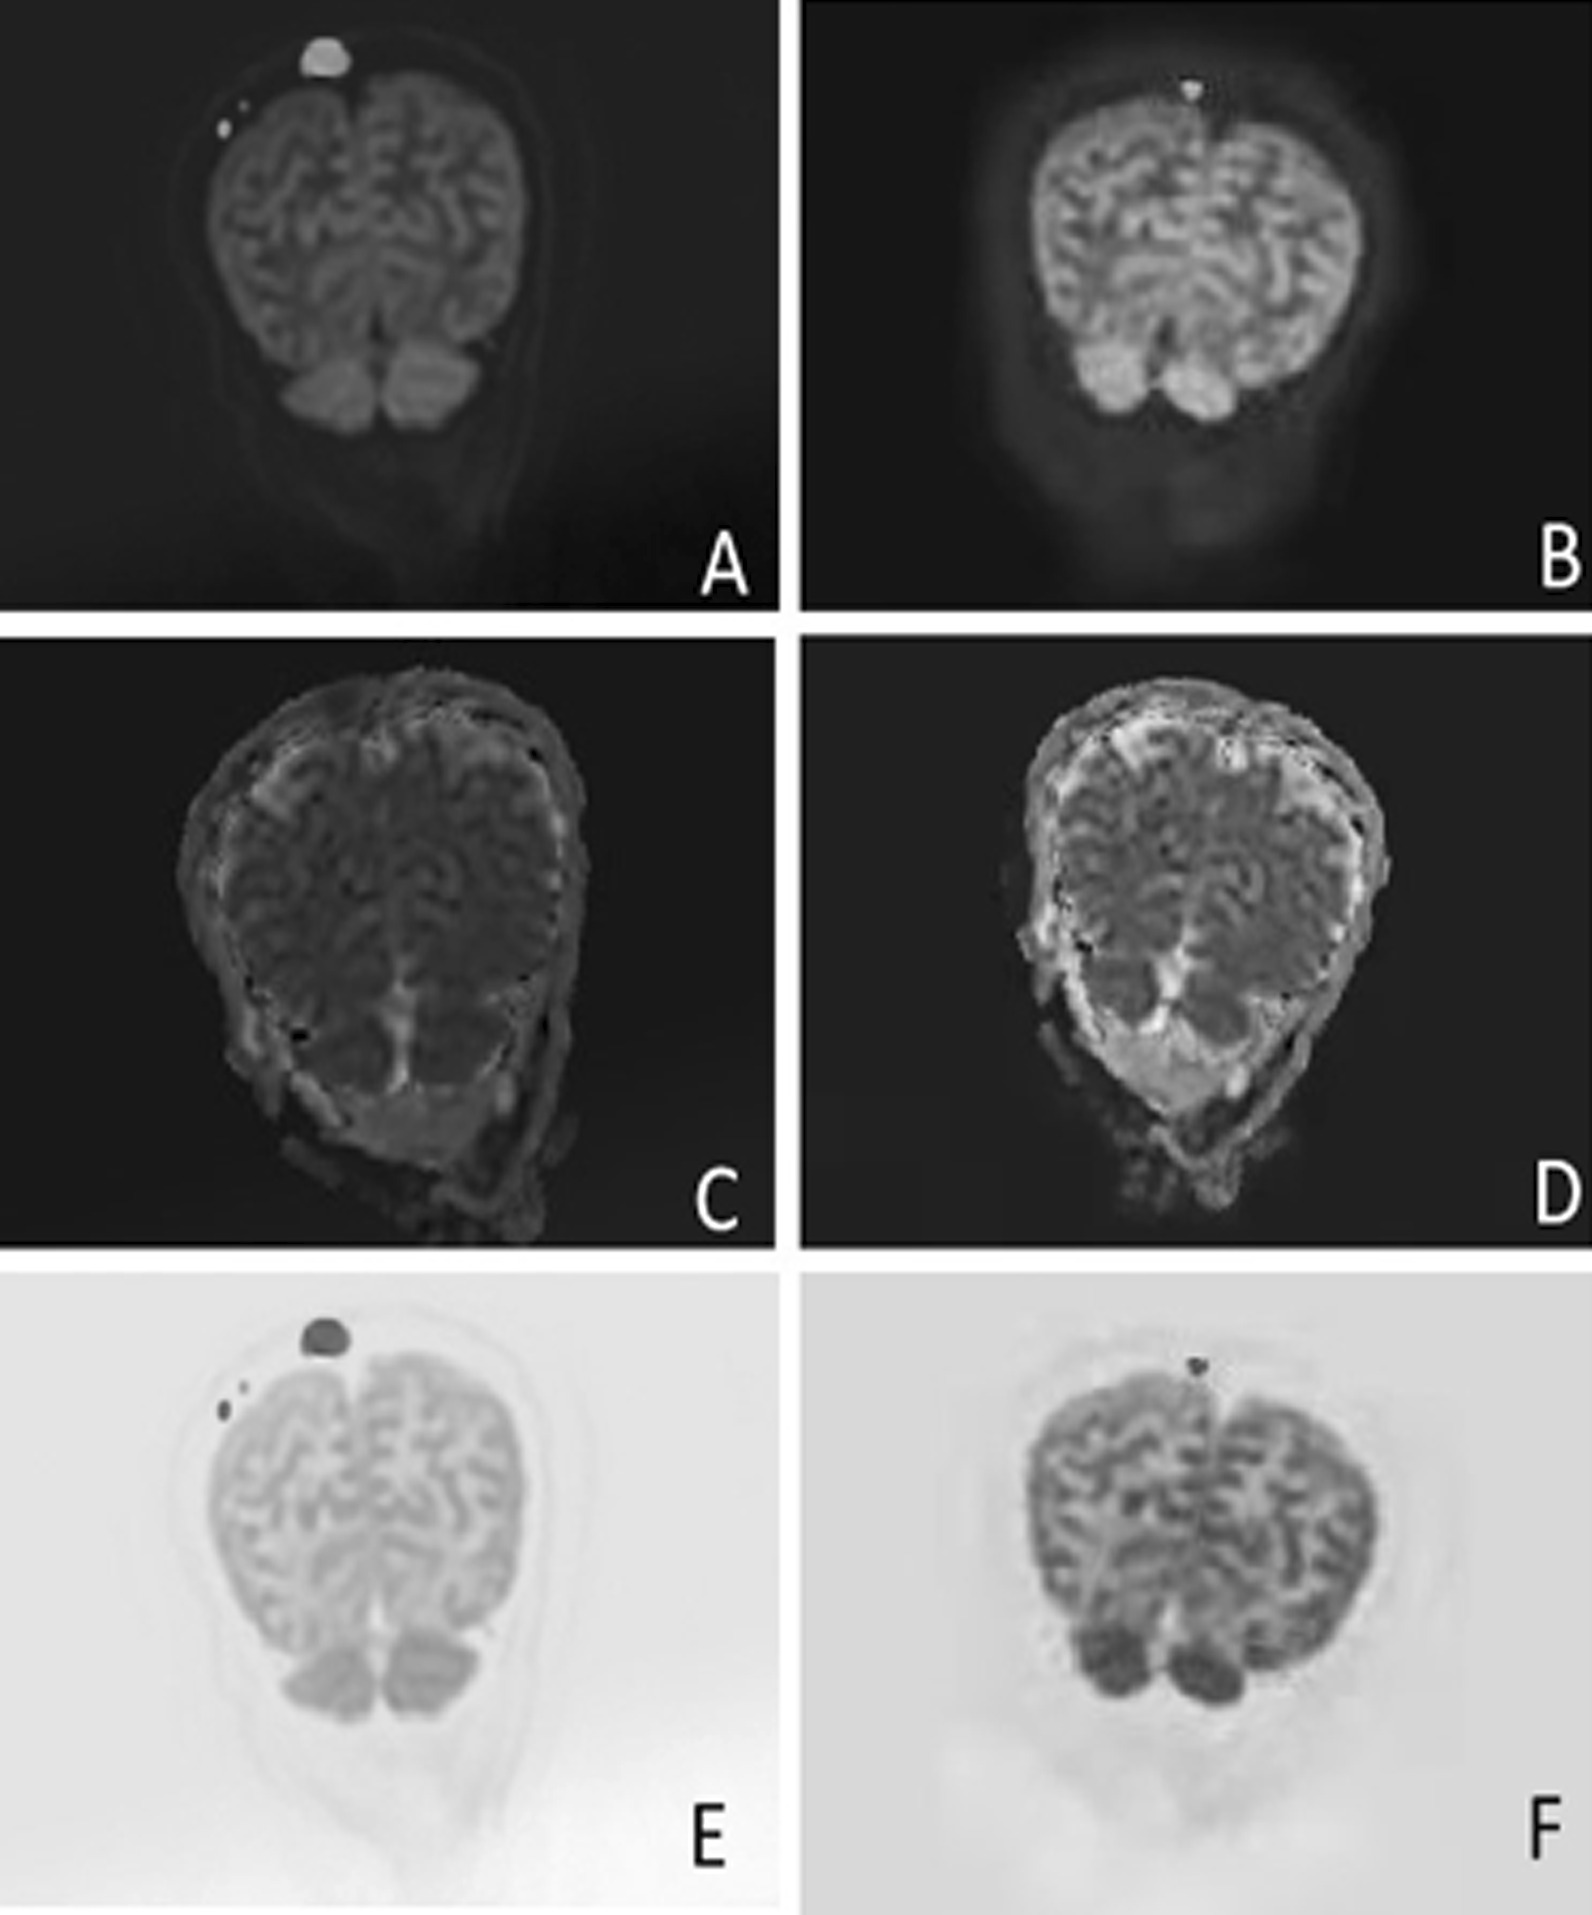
The same patient as Fig. 1, A (DWI b = 1000 s/mm^2^), C (ADC map) and E (inverted MIP image) show MM lesions of skull at baseline visit. B (DWI b = 1000 s/mm^2^), D (ADC map) and F (inverted MIP image) show MM lesions after 4-course chemotherapy. The ADC value of the large lesion in right parietal bone increased from (0.798 × 10^–3^ mm^2^/s) to (0.833 × 10^–3^ mm^2^/s). The change of lesion sizes are more obvious on inverted images (E before treatment; F after treatment) than that on DWI images (A before treatment; B after treatment)
